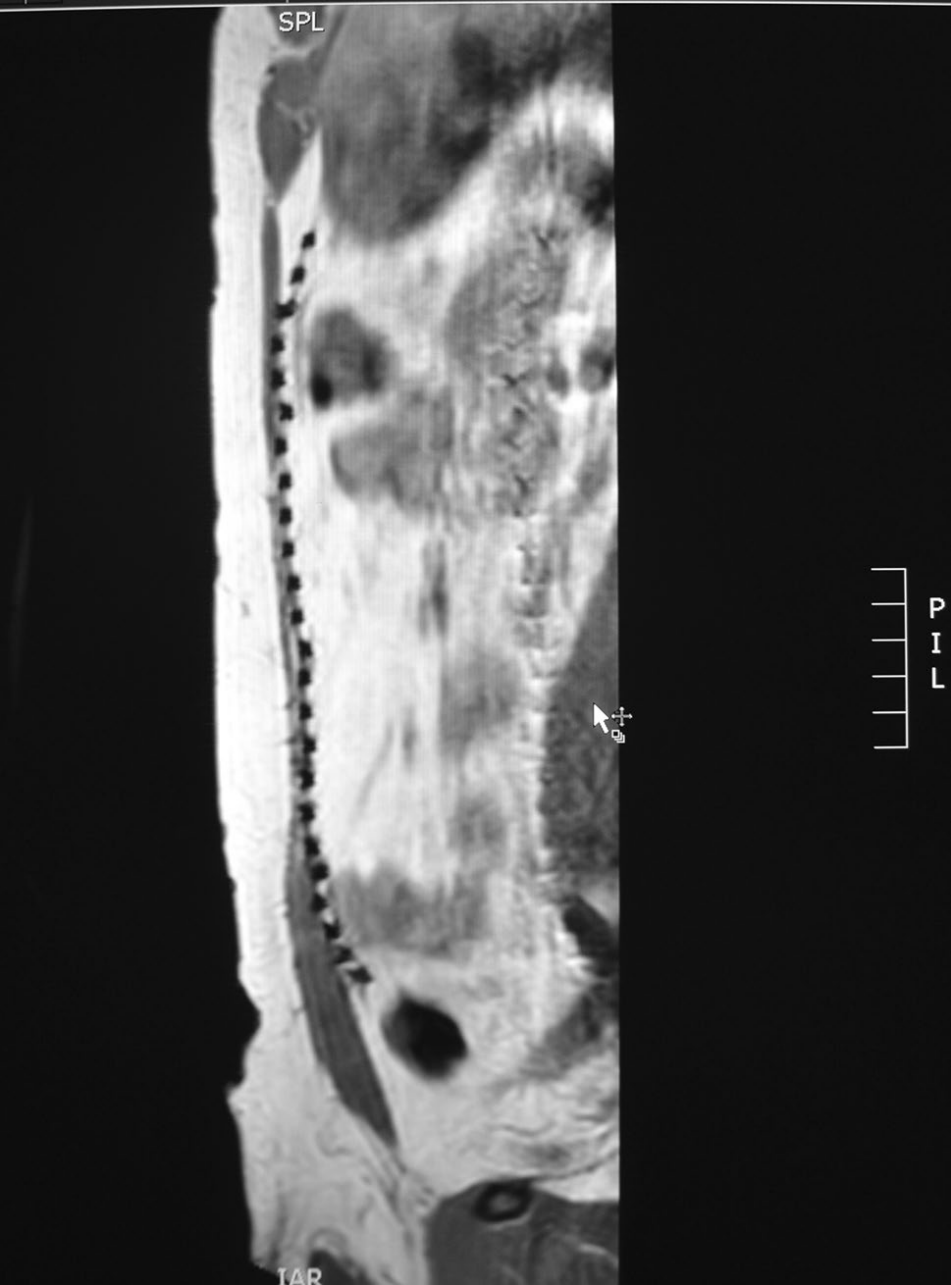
Native sagittal IF FFE (5 mm slice thickness, TE/TR 4.6/329 ms, FA 80°) MRI images after laparoscopic intraperitoneal ventral hernia repair with an iron oxide-loaded PVDF mesh
